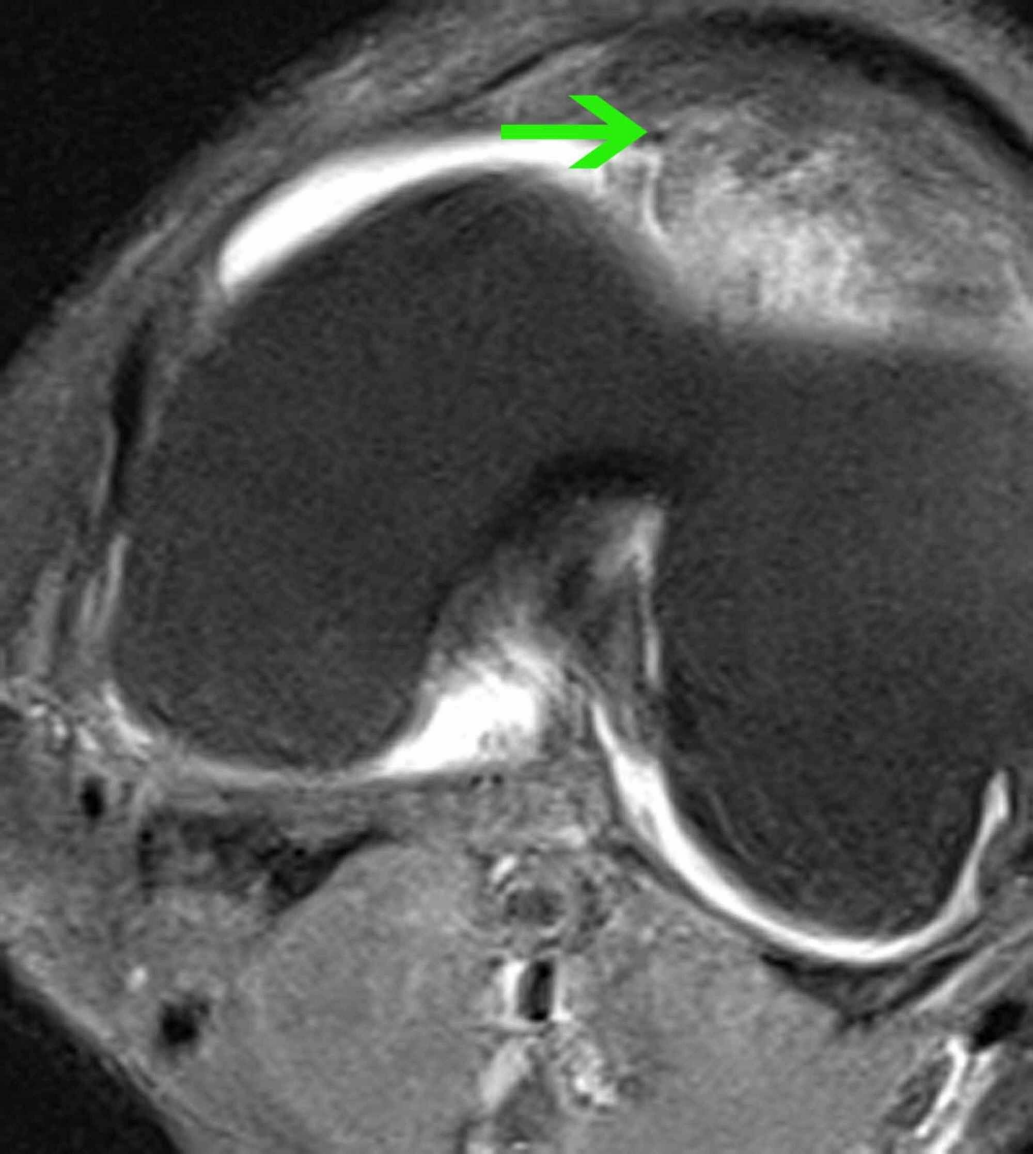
MRI of the left knee (transverse plane) Proton-density turbo spin-echo fat-suppressed (PD TSE FS) sequence, transverse plane, in which the mass is shown to be distal to the level of the patella behind the fat pad and anterior to the lateral femoral condyle (green arrow).
